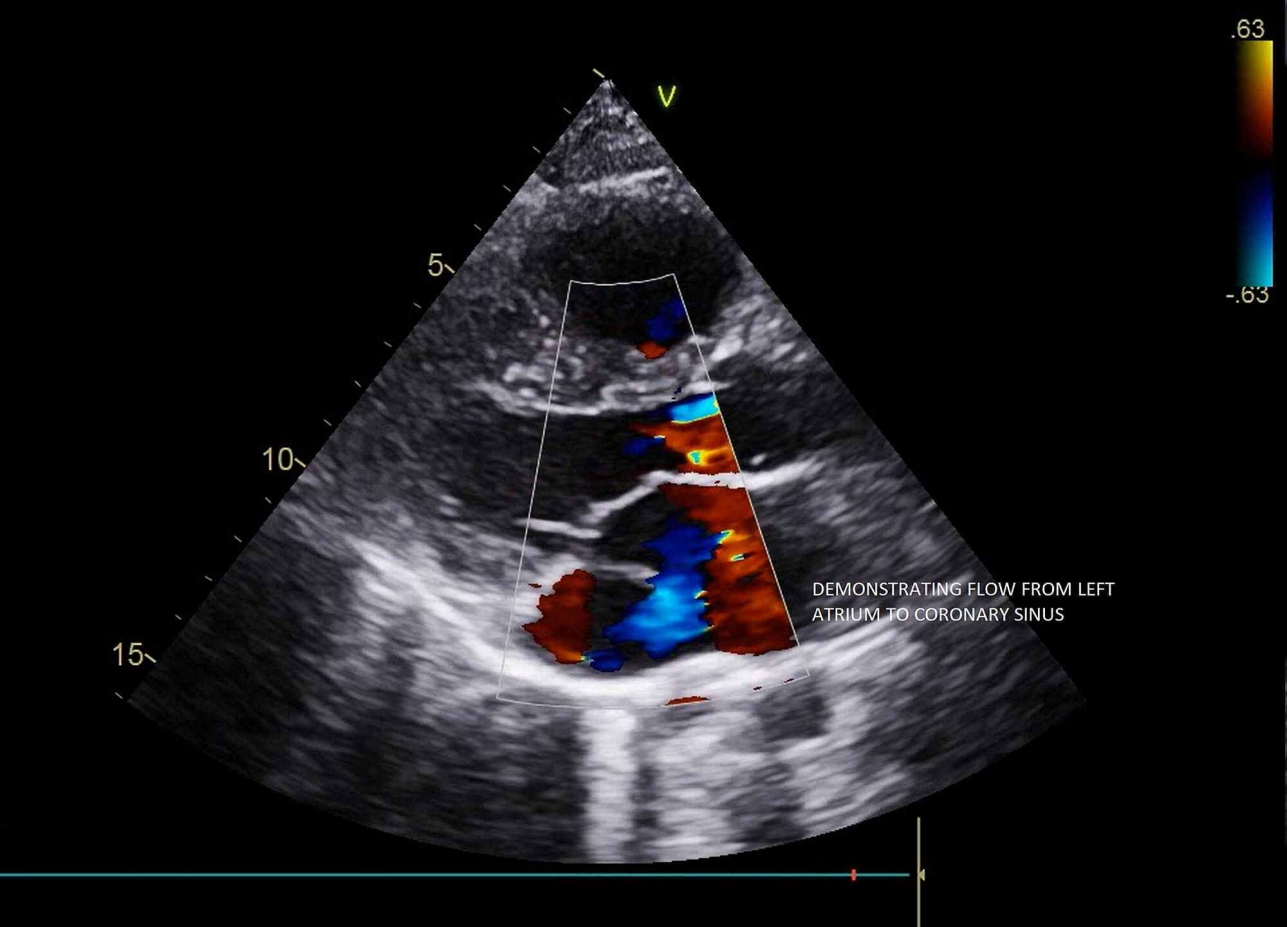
Color Doppler showing flow from the left atrium into the dilated coronary sinus
